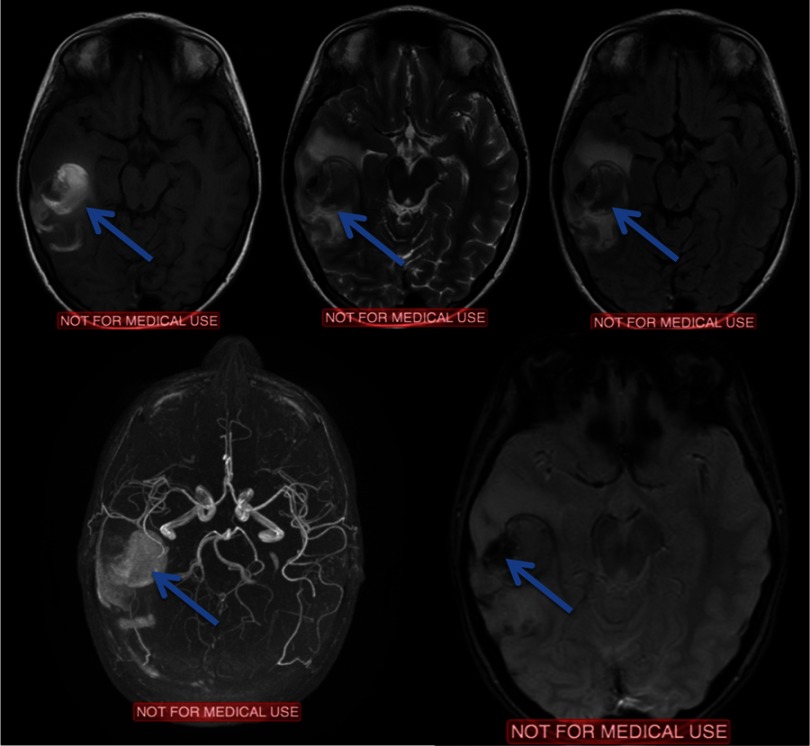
Arrows depict the evolution of signal changes, 10 days after the event. MRI and MRA scan after 10 days. T1W, T2W, FLAIR and T2-GRE images and MRA reconstruction.
